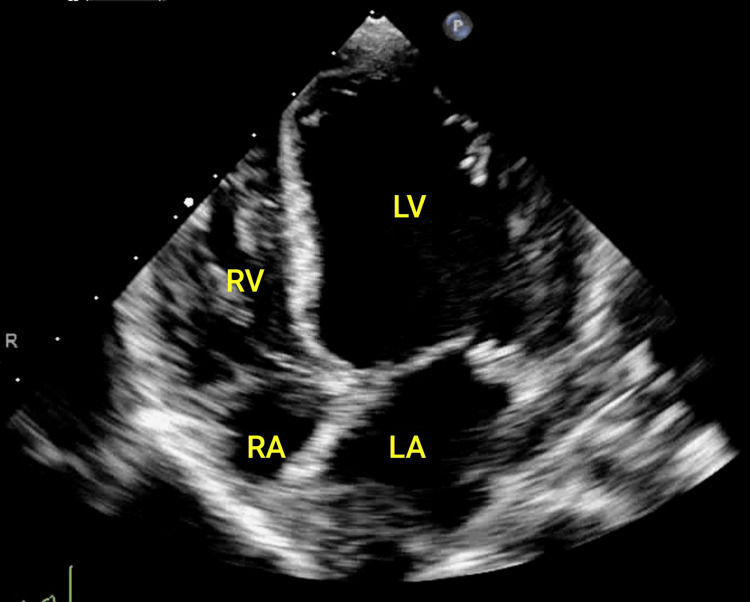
Transthoracic echocardiographic apical four chamber view, showing dilated left ventricle and atrium. LV: Left ventricle, LA: Left atrium, RV: Right ventricle, RA: Right atrium.
